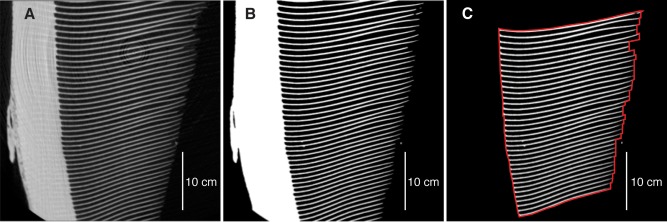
Steps used to calculate plate, IB void, and total volumes in ImageJ. (A) Original CT image. (B) Thresholded image, eliminating grayscale values. (C) Cropped image that includes only complete plates, and removed Zwischensubstanz and bristle clumps. Pixels in white represent the area composed of plate material, and the red outline represents the boundary for measuring total area. IB void area is calculated by subtracting plate area from total area.
